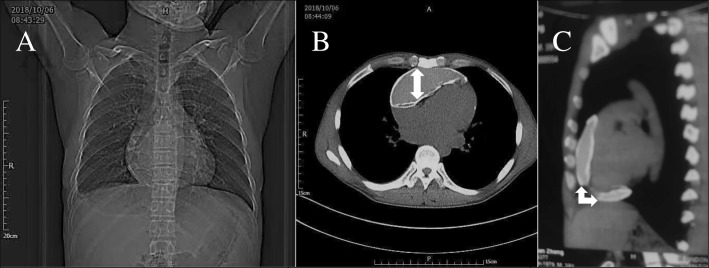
Imaging results, Panel A chest radiography, panels: B & C CTA. Panel A. Chest radiography, Panels B and C CTA with solid arrows demonstrating calcification & fluid
